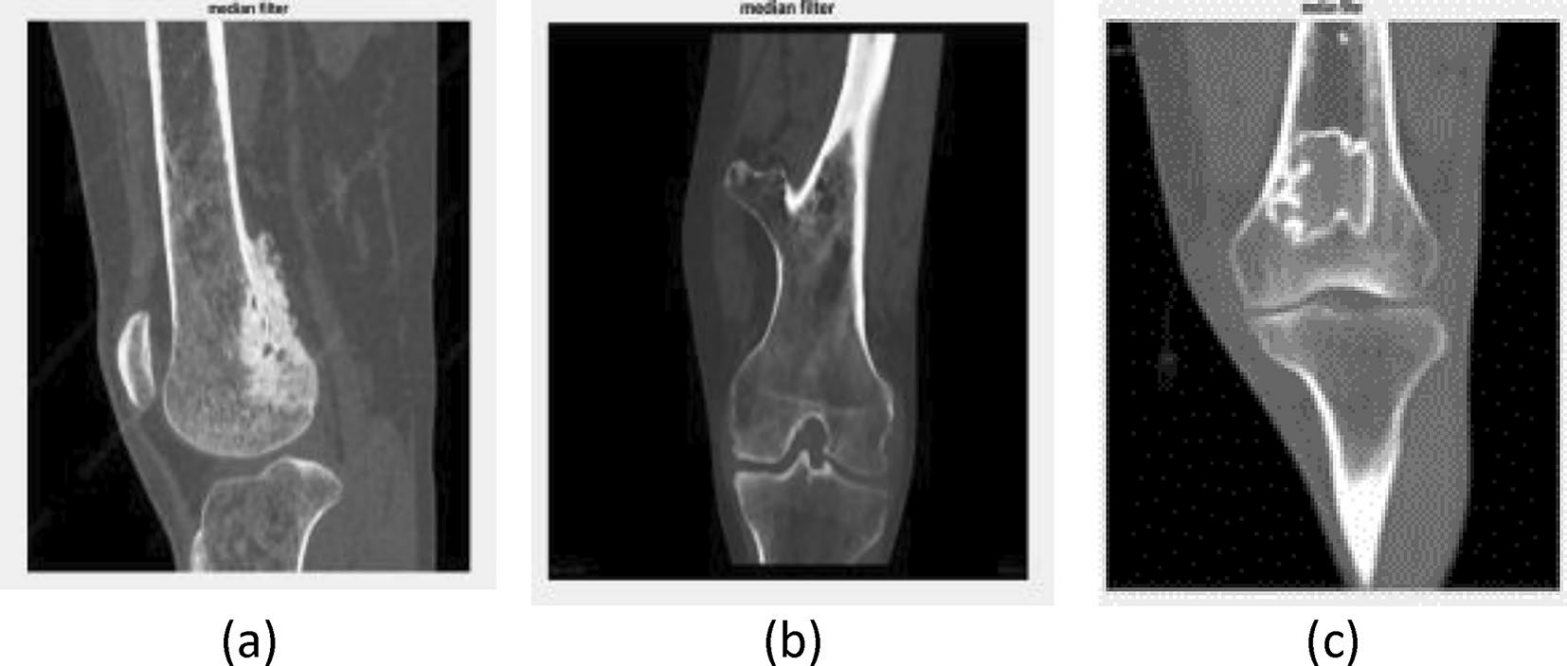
Effect of the median filter: (a) lateral CT of parosteal osteosarcoma, (b) coronal CT of Osteochondroma, and (c) lateral CT of enchondroma.
